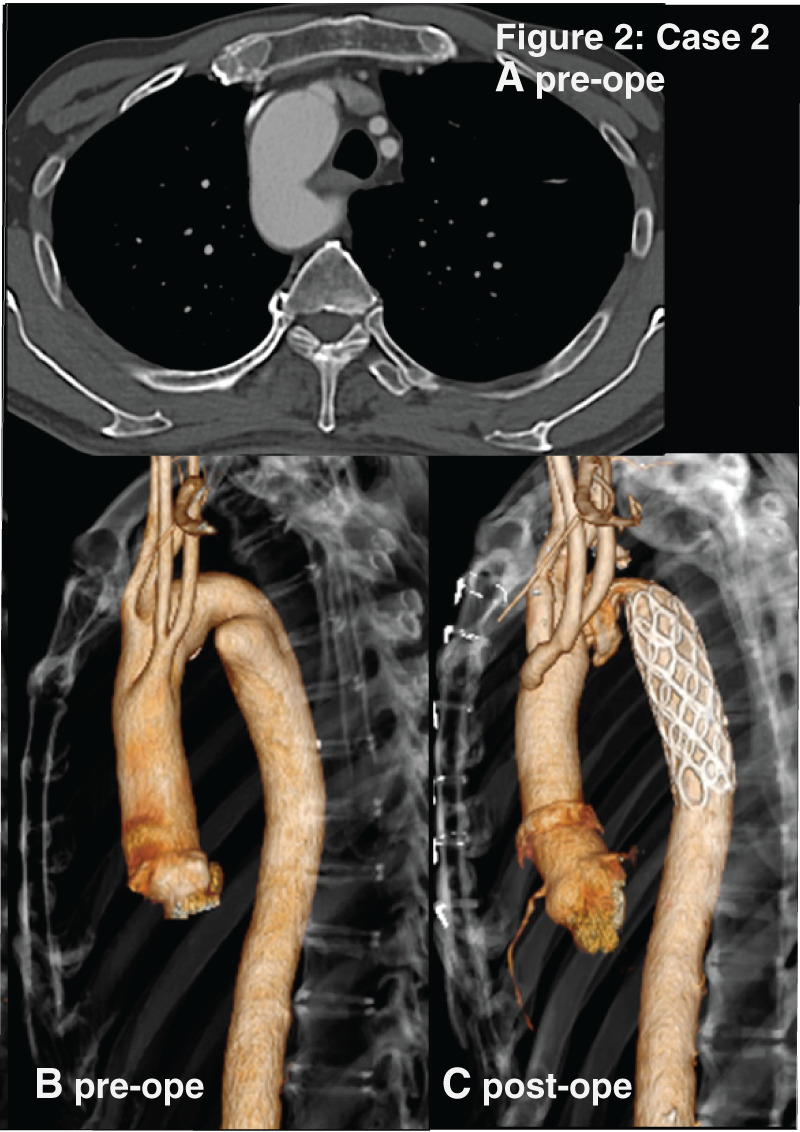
The figure 2 correspond to the images of Case 2 respectively. (A) Preoperative enhanced CT images, axial view. (B) Preoperative 3D-reconstructide CT. (C) Postoperative 3D-reconstructed CT. Case 2 are mirror-image RAAs. RAA, right-sided aortic arch
